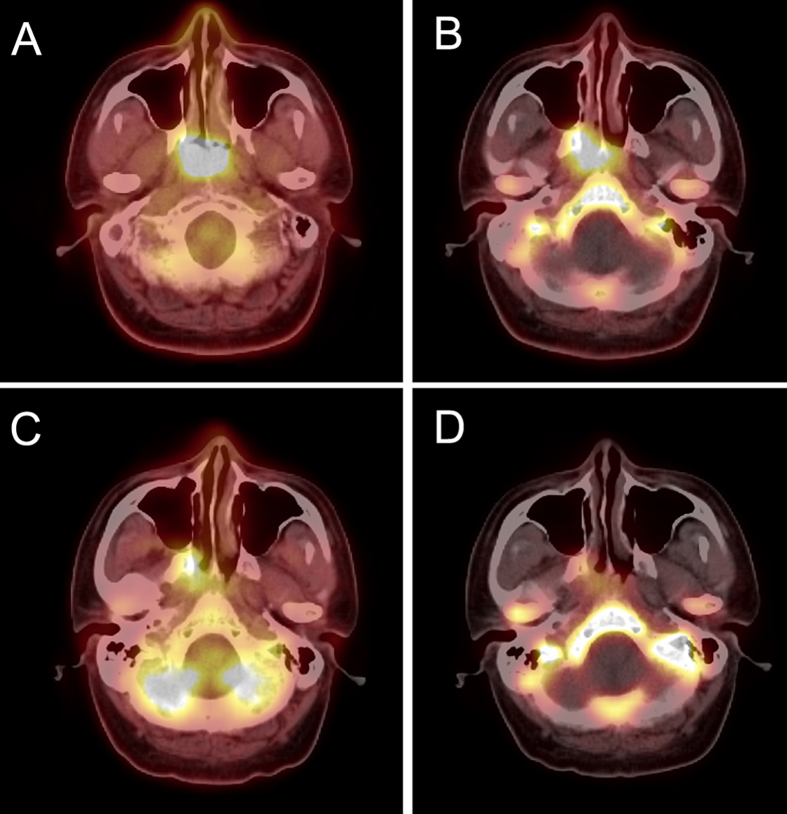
A 36-year-old male NPC patient, we detected high FDG (A) and FLT (B) accumulations in primary lesion, with the SUVmax of 13.1 and 6.5, respectively. We still find obvious uptake of both FDG (C) and FLT (D) after two cycles of NACT, SUVmax = 6.6 and 4.9. At the end of radiotherapy (55 Gy), he reached only PR according to RECIST criteria.
